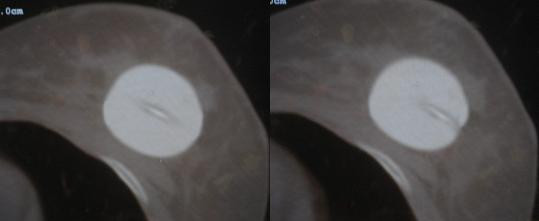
CT scan slices from case 3 showing balloon symmetry.
